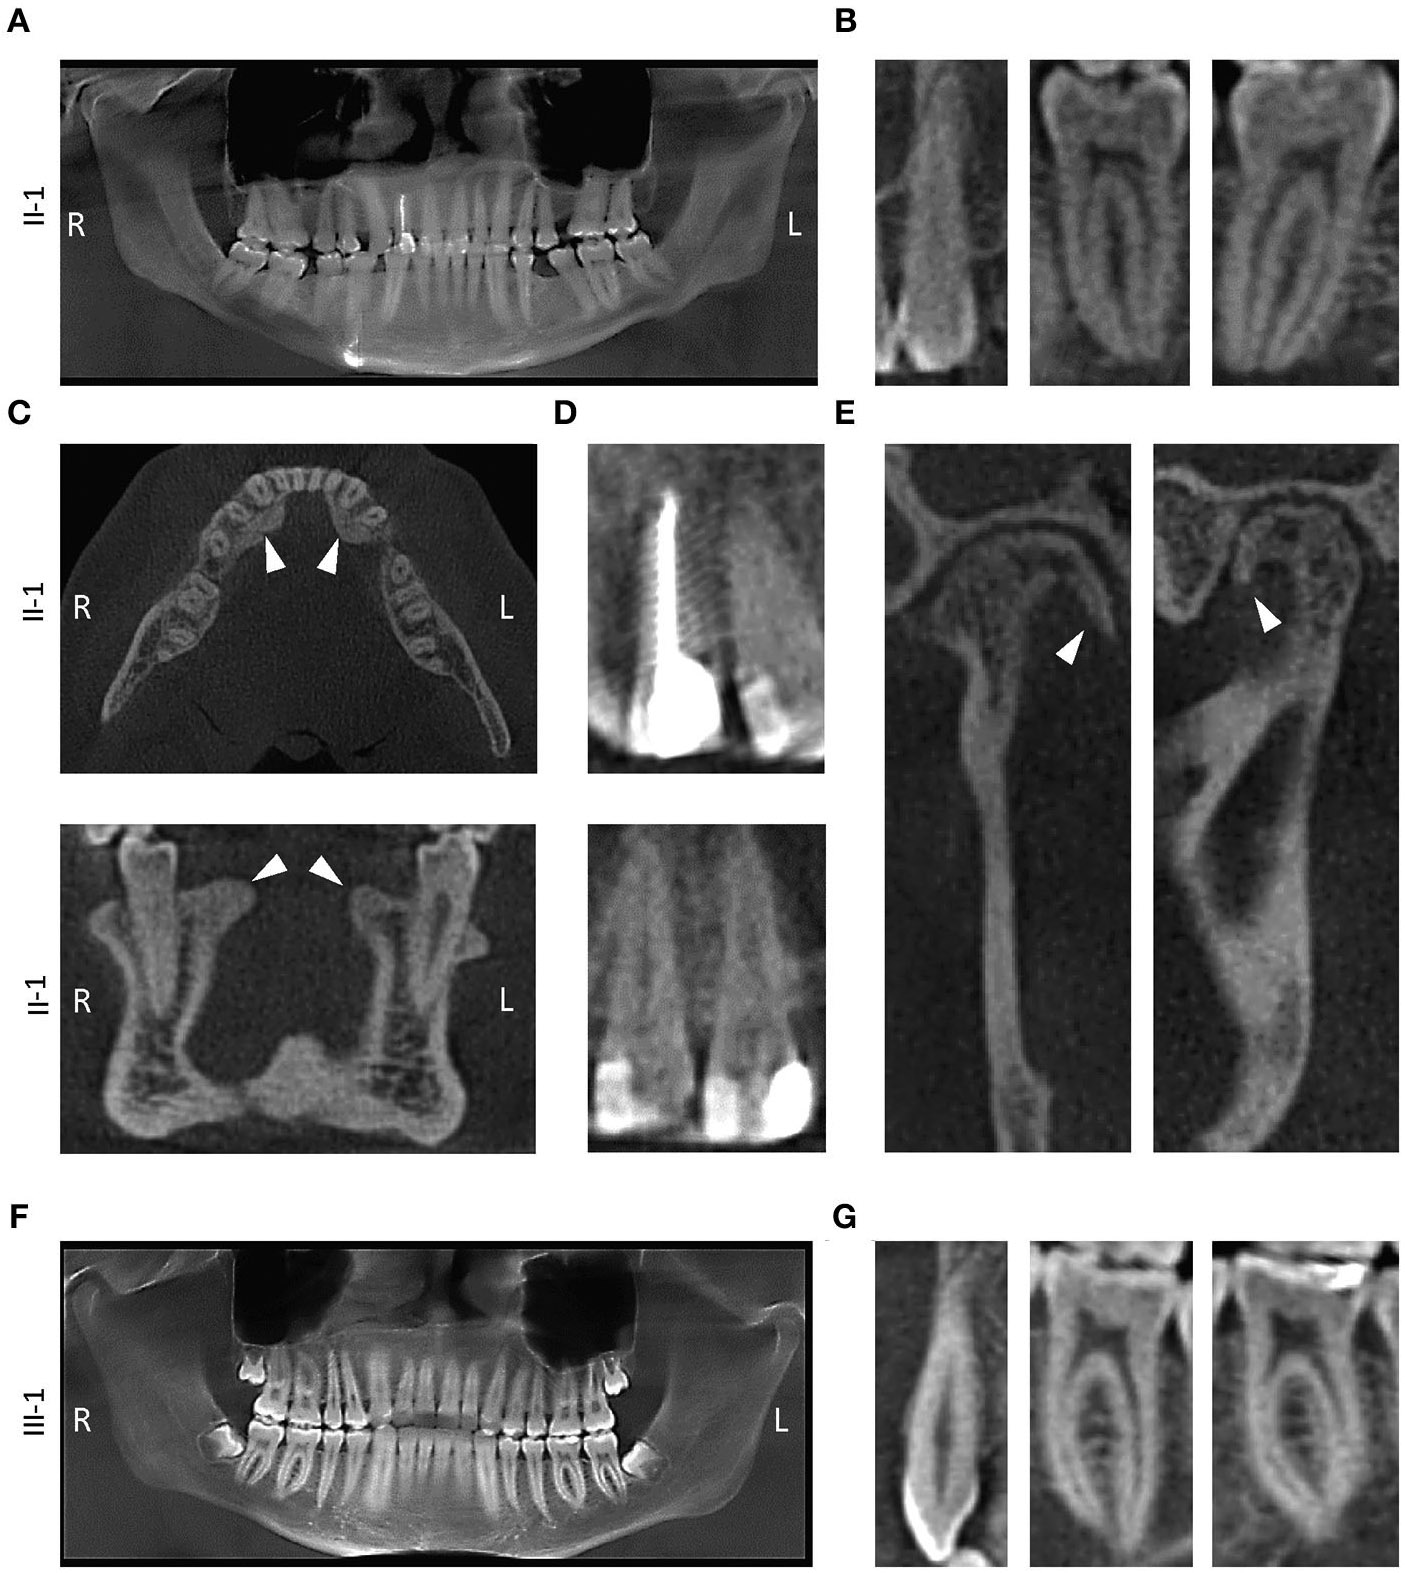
Radiographic findings. (A) Panoramic x-ray from proband II-1 showing thickened dentin and obstructed pulp chambers. (B) 2D slices from II-1 cone beam computed tomography (CBCT) exam showing tooth # 11, 21, and 30 with constricted pulp canals. (C) Transverse (top) and a coronal (bottom) slice of the mandible showing lingual tori (white arrowheads). (D) Restorations in the teeth of proband with a root canal on tooth #7 (top) and class V restorations with teeth # 8 and 9 (bottom). (E) Coronal (left) and a sagittal (right) slice of the right mandibular condyle showing osteophytes (white arrowhead). (F) Panoramic x-ray from III-1. (G) 2D slices showing tooth # 11, 21, and 30.
